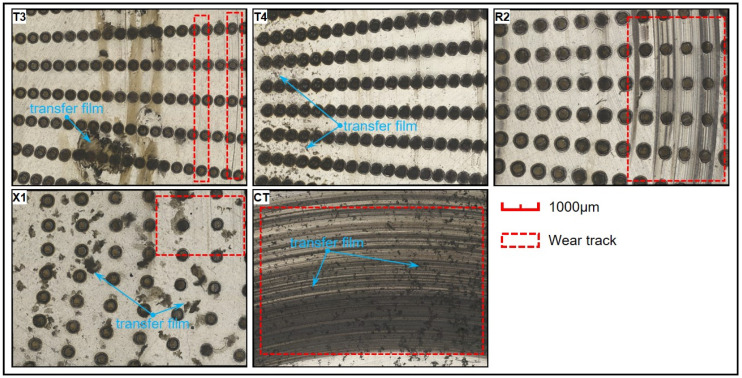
Representative wear morphologies of the 1040 steel discs with PEEK transfer films before ultrasonic washing.
